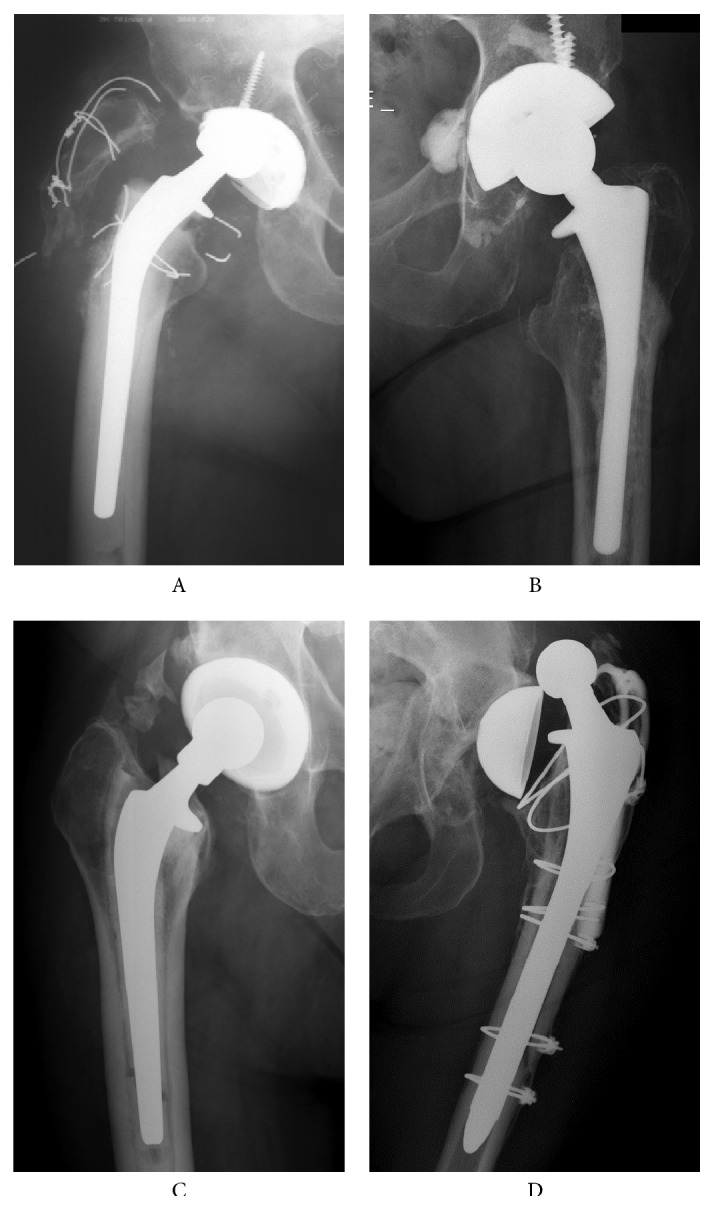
Radiographs from patients from whom heads A–D were retrieved. Radiographs of head E were not available.
